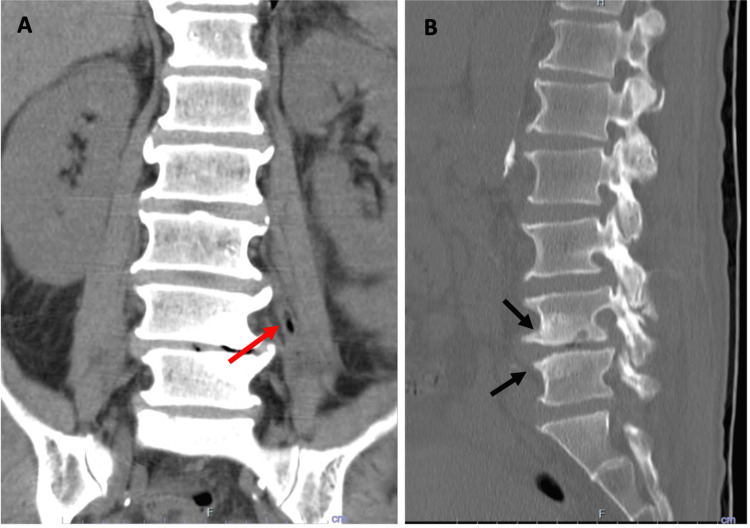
Non-contrast lumbar CT A - coronal view showing the presence of intramuscular air, suggestive of an infectious process (red arrow); B - sagittal view, densification of the bone structure surface (black arrows). CT - Computed tomography
